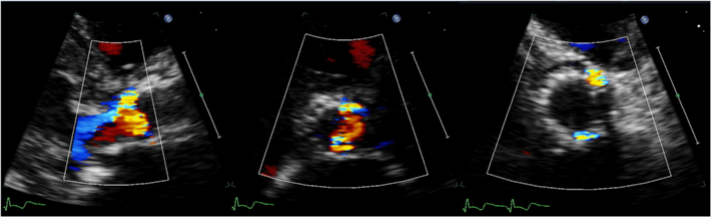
Parasternal long-axis showing two jets (*left*). Short-axis colour Doppler evaluation of PVL should start just below the valve stent (*middle*) *but should always be followed by* scanning more cranial to better detect the “origin” or “neck” of the PVL (*right*)
